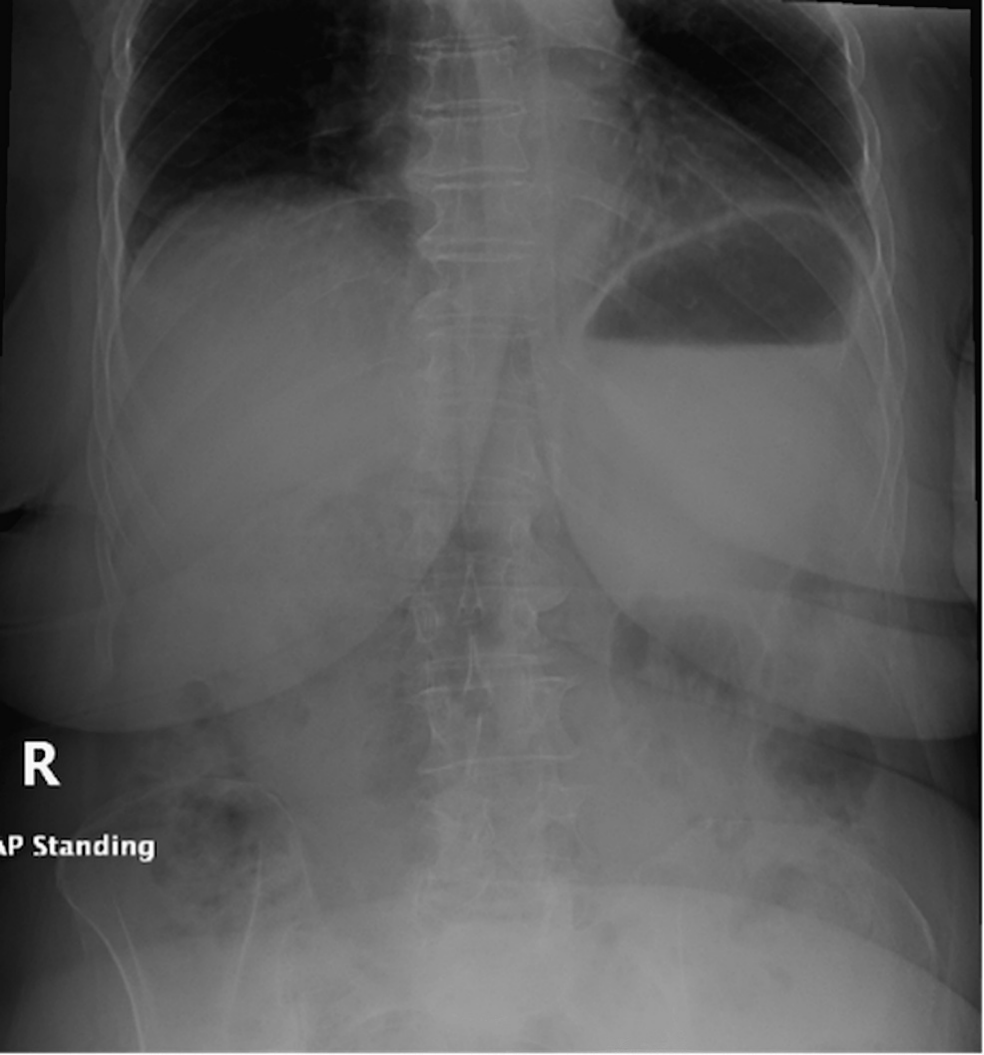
Erect abdomen X-ray showing no air under the diaphragm.
